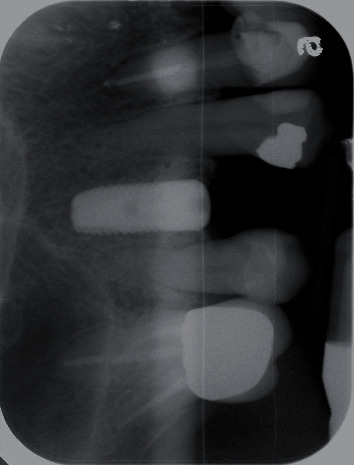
X-ray following the implant placement.
